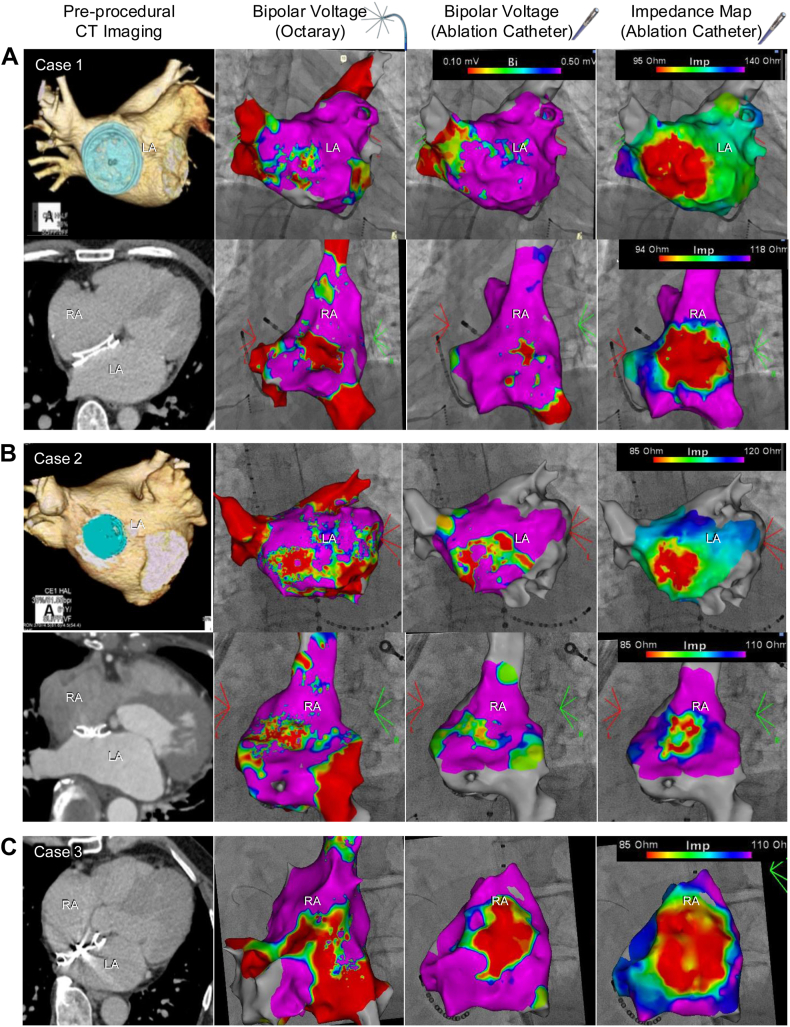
Voltage and impedance mapping of atrial septal occluder devices. Panels A–C correspond to cases 1–3. For each case, preprocedural imaging is shown in the left column, followed by representative bipolar voltage maps and a generator-impedance map acquired using the multielectrode catheter and RF ablation catheter. Bipolar voltage maps demonstrate heterogeneous and fragmented low-amplitude distributions over the occluder surface, whereas generator-impedance maps delineate a contiguous region closely matching the expected device footprint. CT = computed tomography; LA = left atrium; RA = right atrium.
